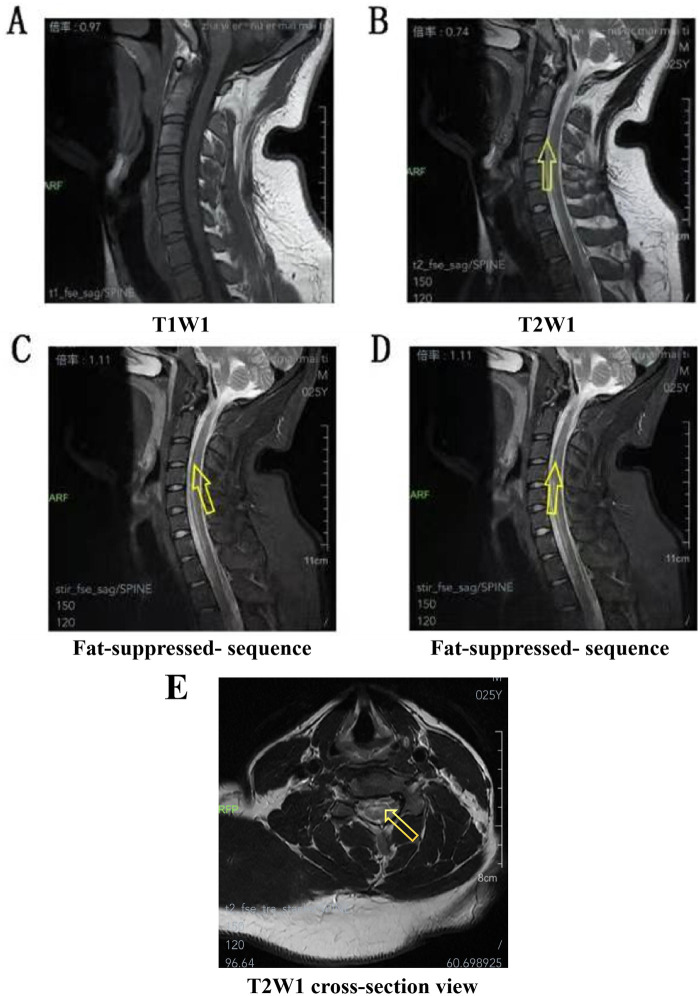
Spinal cord MRI. (A) No abnormal signals were observed in T1WI. (B) On T2WI, abnormal signals can be observed at the anterior margin of the spinal cord at the level of C4-C1 vertebrae. (C,D) Abnormal signals at the anterior margin of the spinal cord at the level of cervical 4 to thoracic 1 vertebra can be observed in the fat-suppression sequence. (E) On the T2WI sagittal view, abnormal signals in the spinal cord at the level of the C4-C5 intervertebral disc.
